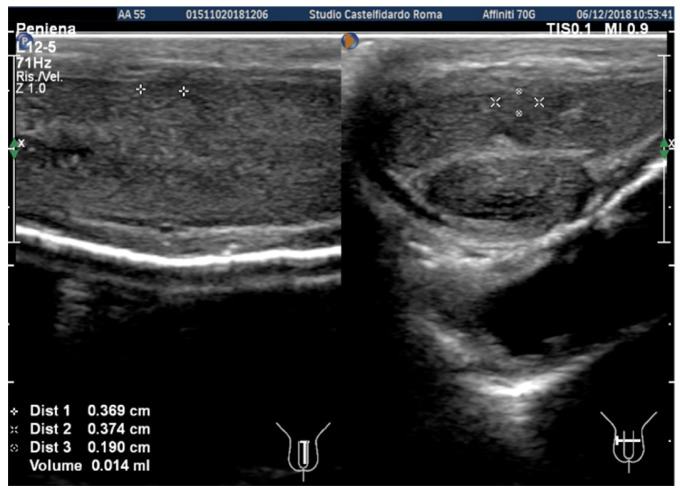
Ultrasonography of the penis after the third treatment cycle (longitudinal and transverse views).
